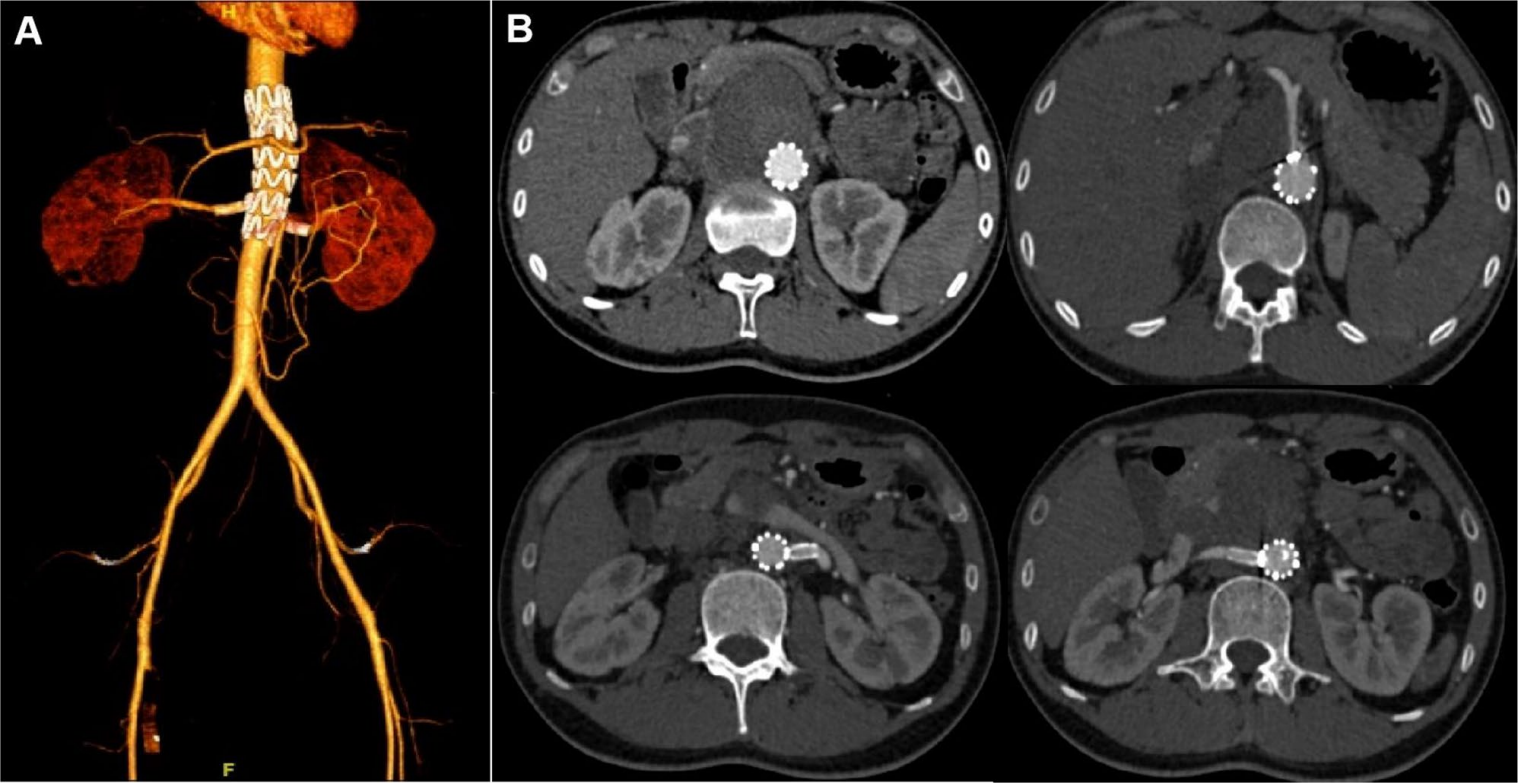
Follow-up CTA at 18 months with volume rendering reconstructions (A) and axial views (B) showed the aneurysm was completed excluded and the patent visceral arteries
